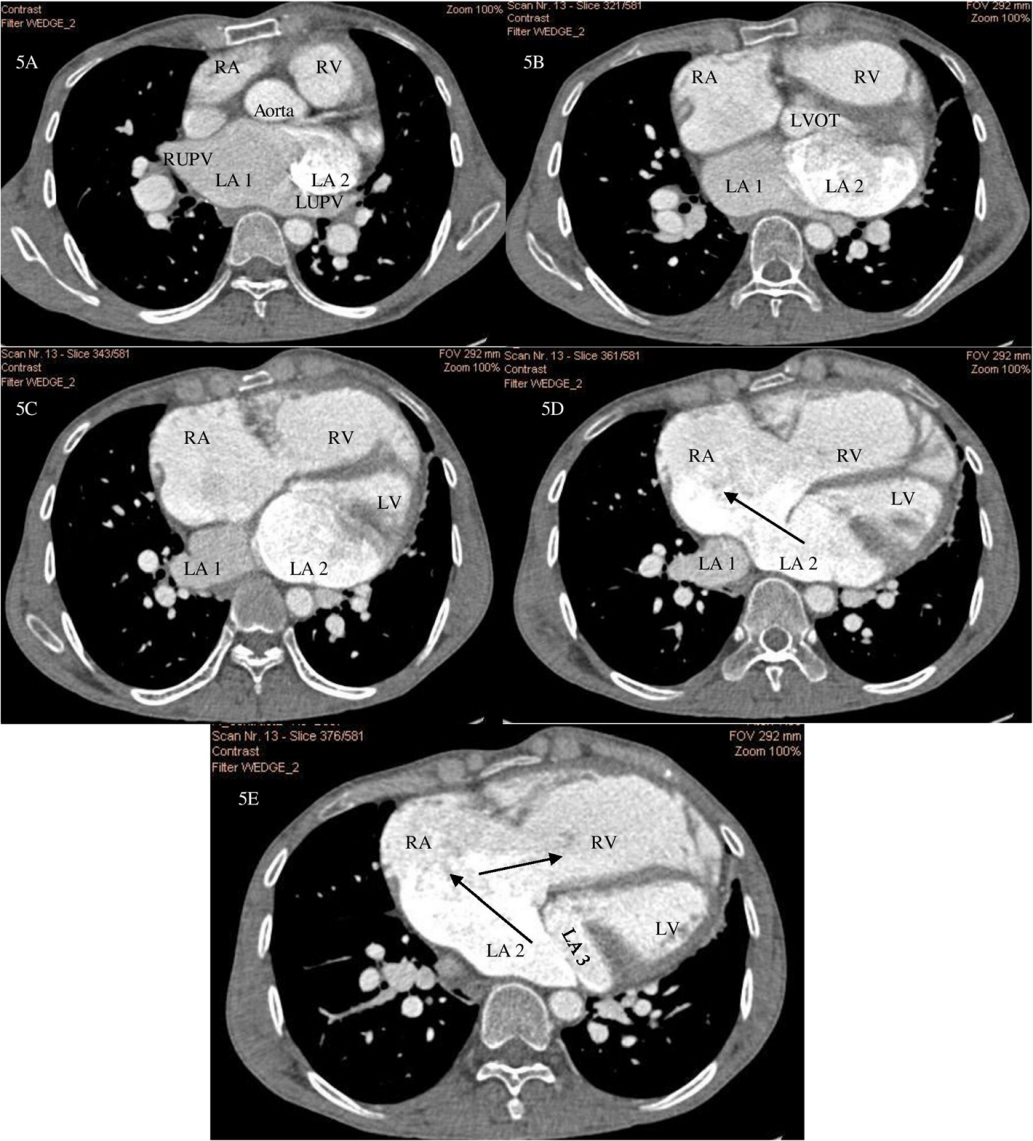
A, B, C, D, E Cardiac CT with contrast injected in left antecubital vein showing appearance of contrast firstly into middle chamber of LA (LA 2) (A) then progressing to RA through ASD (arrows in D and E) suggestive of PLSVC draining into LA due to completely unroofed coronary sinus confirming presence of Raghib syndrome. Three chambers of LA (labelled LA1, LA 2, and LA3) are clearly visualised. RA, right atrium; LA, left atrium; RV, right ventricle; LV, left ventricle; RUPV, right upper pulmonary vein; LUPV, left upper pulmonary vein; LVOT, left ventricular outflow tract
